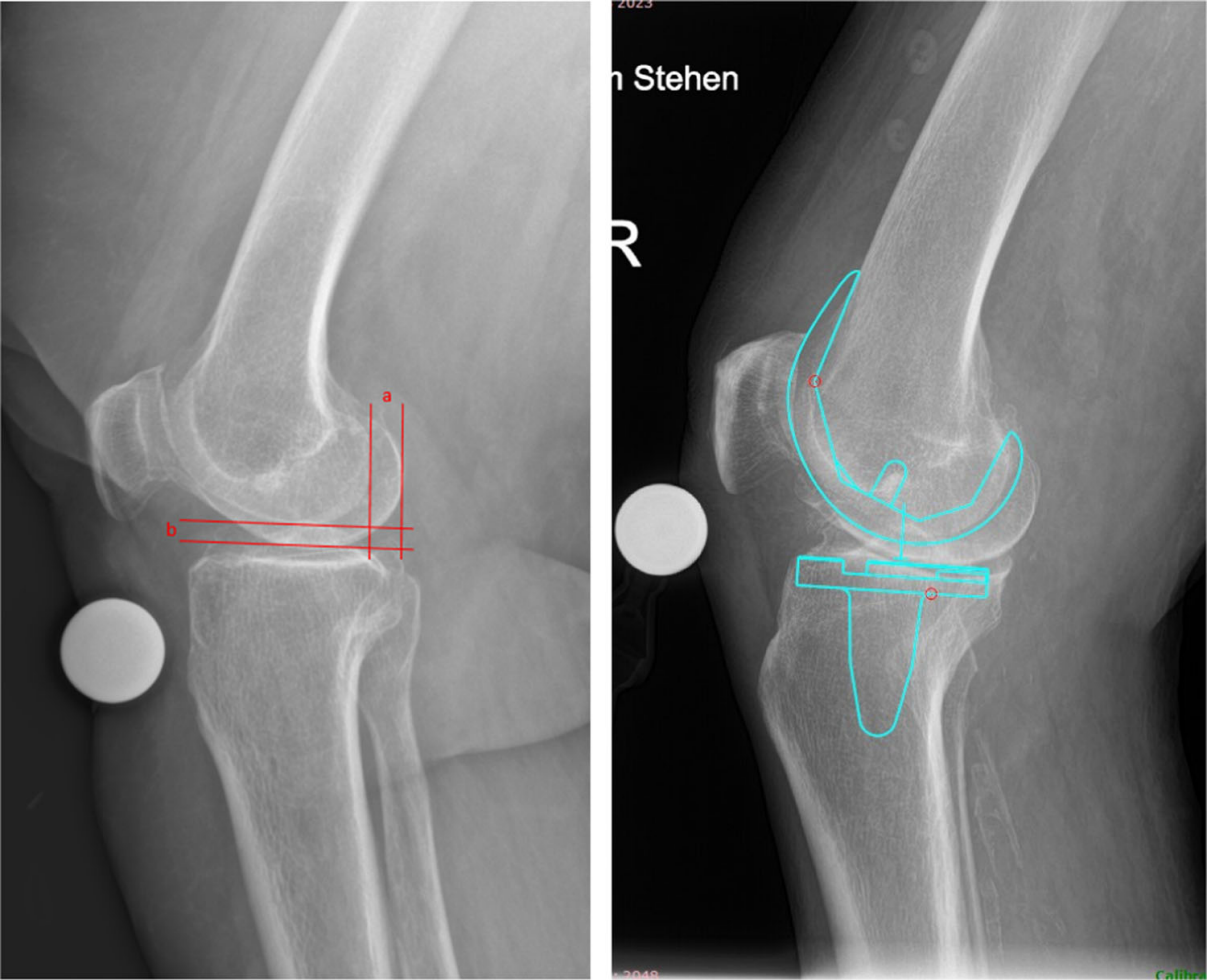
Left Lateral knee radiograph showcasing measurements of quality criteria. a—knee rotation, mm. b—knee abduction/adduction, mm. Right Lateral knee radiograph showcasing digital templating using TraumaCad (Version 2.5; Brainlab Ltd.).
